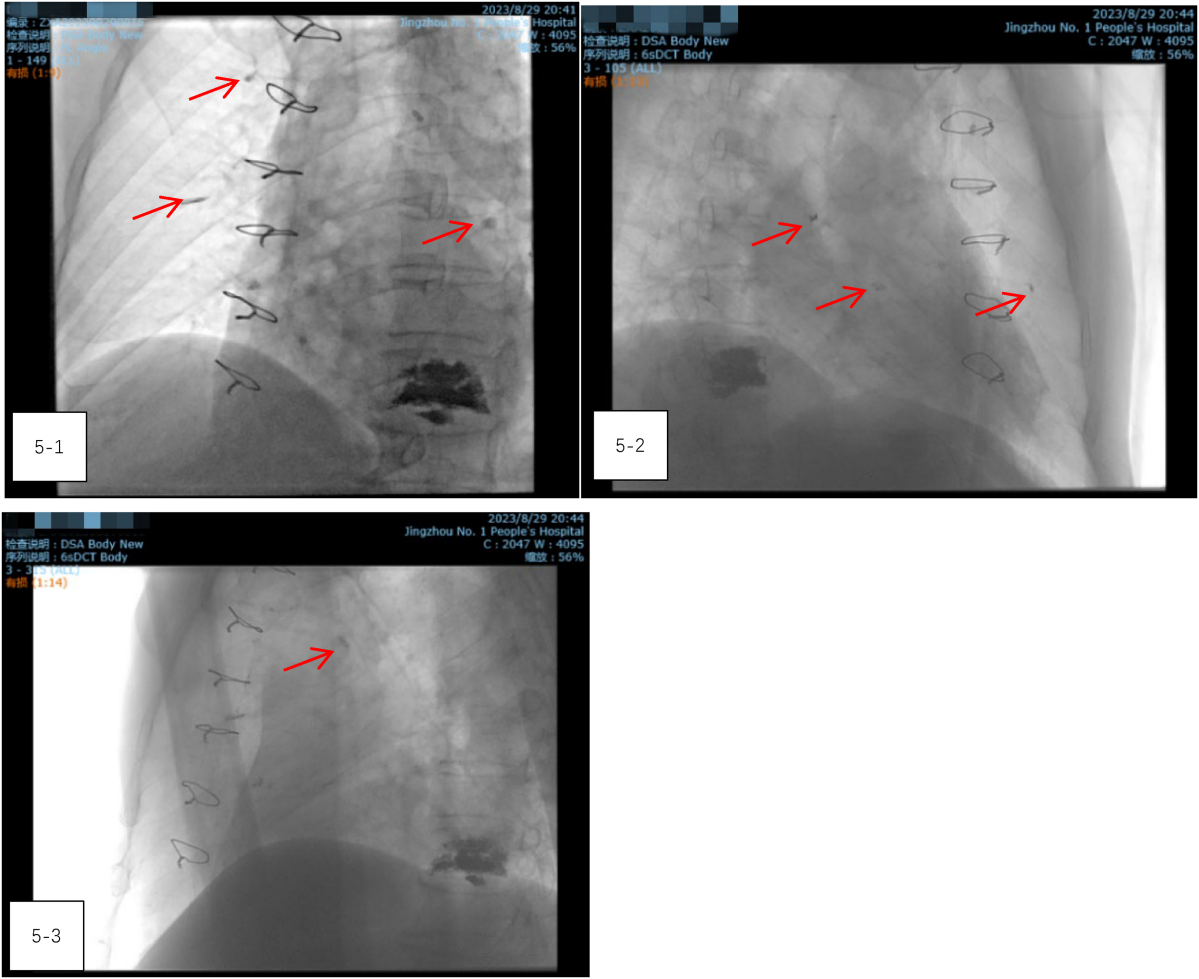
(5-1–5-3) Radiography showed a reduction in the size of the bone cement within the heart, but an increase in emboli within the pulmonary artery.
